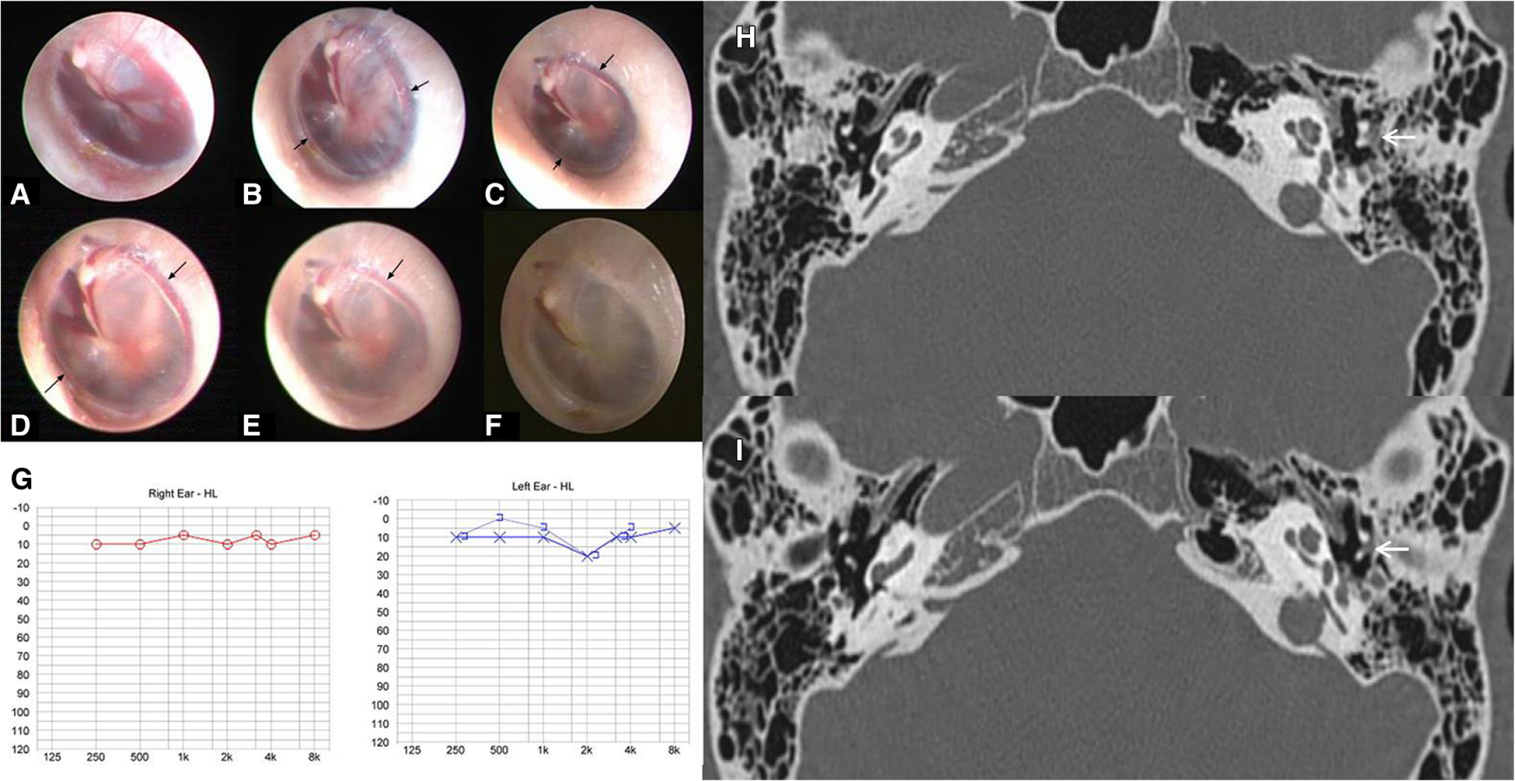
Serial otoendoscopic findings in patient 1. Note that minor bleeding is visible behind the intact left tympanic membrane (TM) and along the line between the annular ligament and epidermis of the TM (black arrows in panels (a - e). a Day 0, (b) Day 1, (c) Day 2, (d) Day 3, (e) Day 4, (f) Day 15. Pure tone audiometry tested the day of trauma (Day 0) revealed a minimum air-bone gap on the left side (g) which was improved after 1 month (h). The axial views of temporal bone computed tomography at Day 0 revealed a very small soft tissue density in the Prussak’s space (white arrows), consistent with iTM hemorrhage observed on otoendoscopic examination (i and j)
